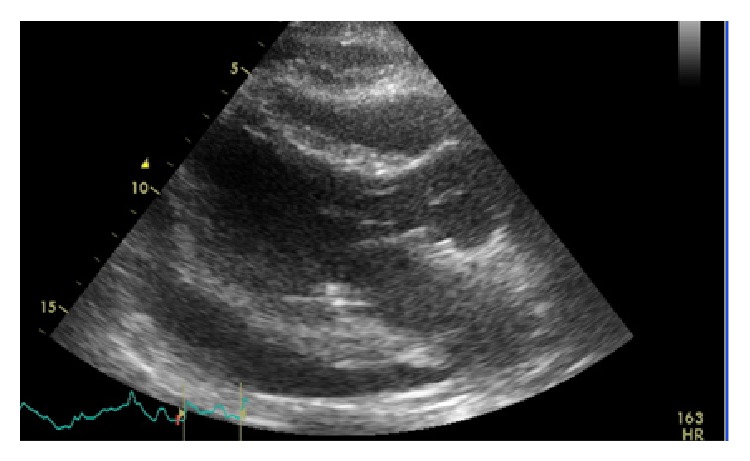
Echocardiographic image showing collapsed right ventricle with effusion.
